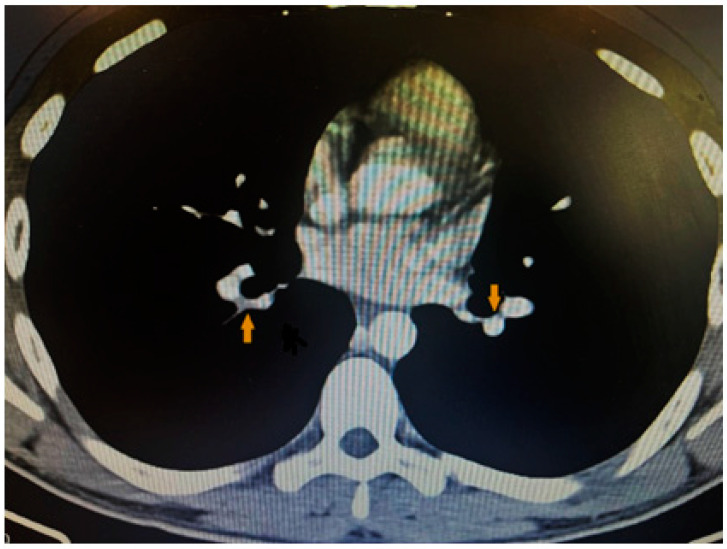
CT showing filling defects within the pulmonary vasculature with acute pulmonary emboli.
